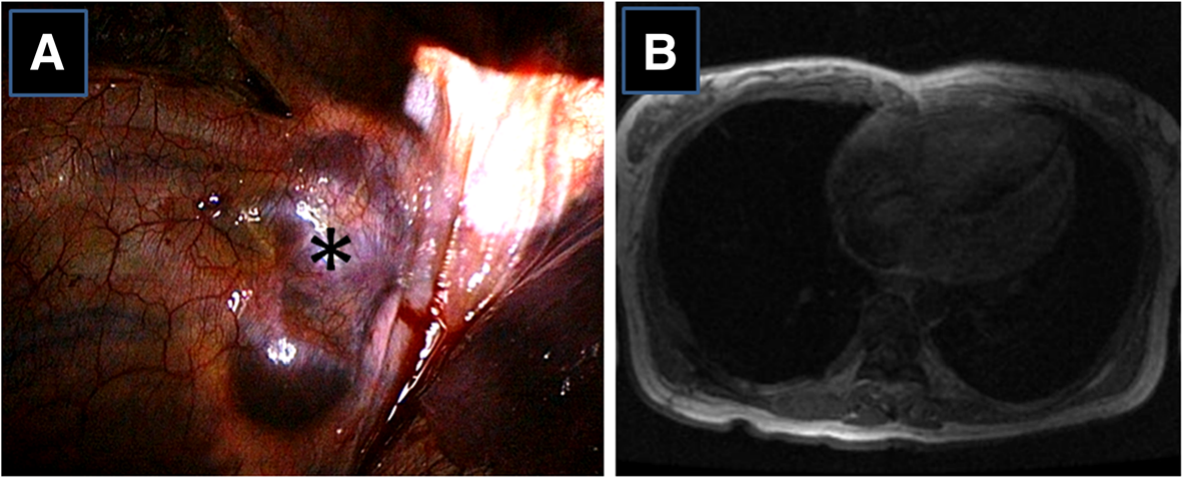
Intraoperative photo and postoperative image. A) Intraoperative photo showing a well-demarcated, dark-coloured mass (* = extramedullary haematopoietic tumour). B) MRI of the chest, obtained 7 months after surgery, revealed no local recurrence.
